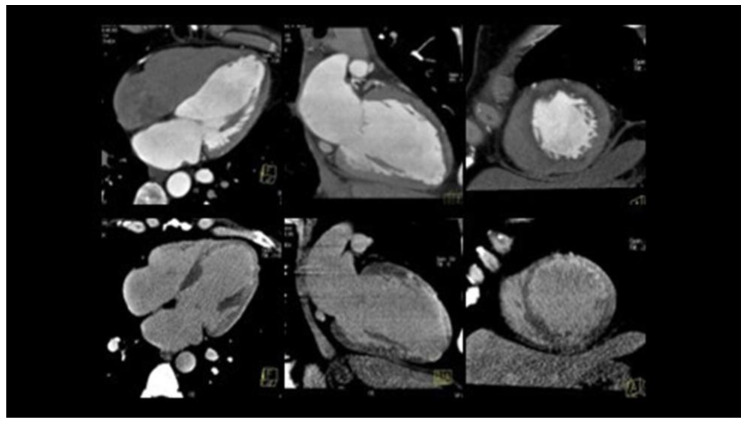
Cardiac CT of the same patient in Figure 4, showing contrast enhancement of the LV anterior wall, secondary to myocardial infarction. CT: computer tomography; LV: left ventricle.
